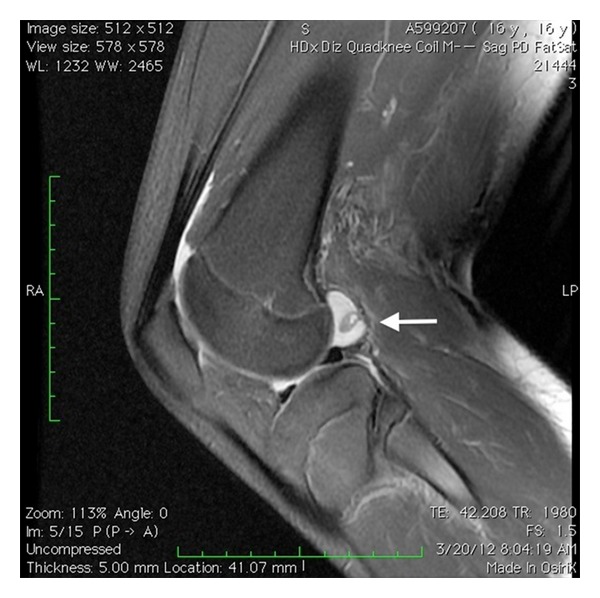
Sagittal MRI view of knee, demonstrating edema around the popliteus tendon.
